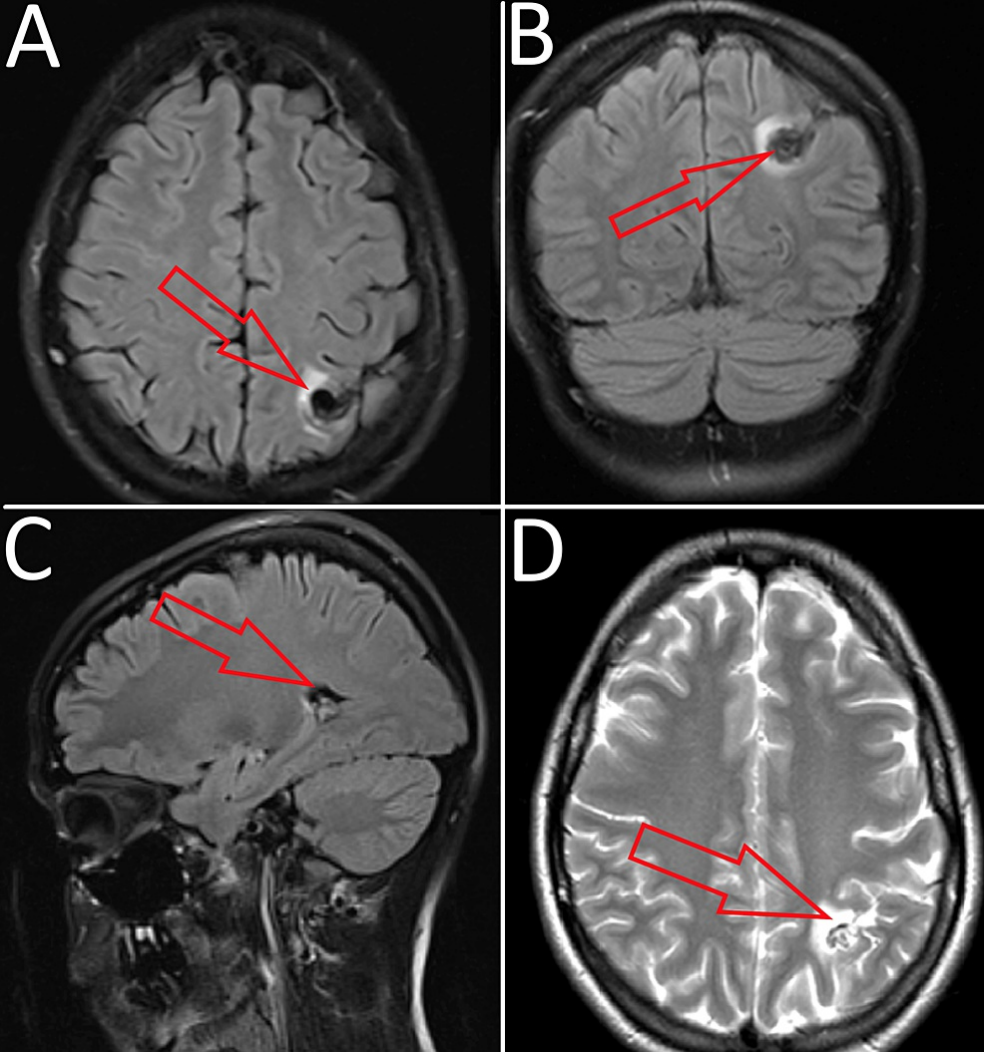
Preoperative MRI showing the location of the cAVM within the left intraparietal sulcus (A) Т1-weighted image from the axial plane; (B) T1-weighted image from the coronal plane; (C) T1-weighted image from the sagittal plane; (D) T2-weighted image from the axial plane cAVM: cerebral arteriovenous malformation
